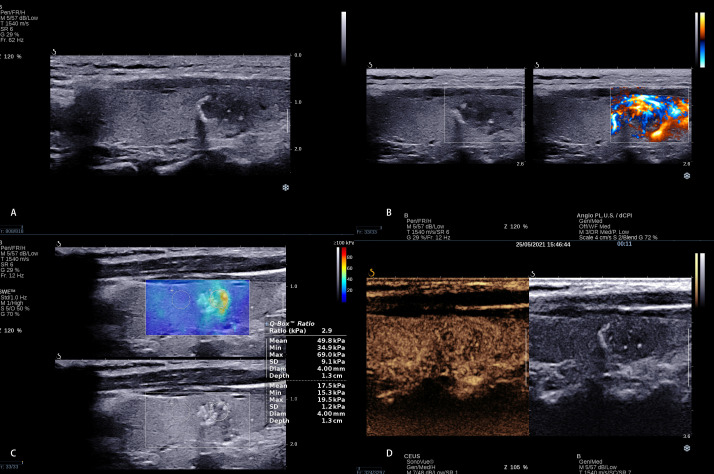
A 13 × 7 mm thyroid nodule in the right lobe of a 31-year-old woman. (A) Conventional ultrasound showed a solid hypo-echoic, ill-defined nodule with calcifications; the shape was wider than taller and the contact extent with adjacent thyroid capsule <25%. (B) AP shows that the vascularization of nodule could be classified as Grade II. (C) SWE measurement shows that Emin, Emean, and Emax were 34.9 kPa, 49.8 kPa, and 69.0 kPa, respectively. (D) CEUS shows continuous capsule at CEUS. The calculated predictive value with the logistic regression formula was 0.673 (<2.0805), which predicted no CLNM.
